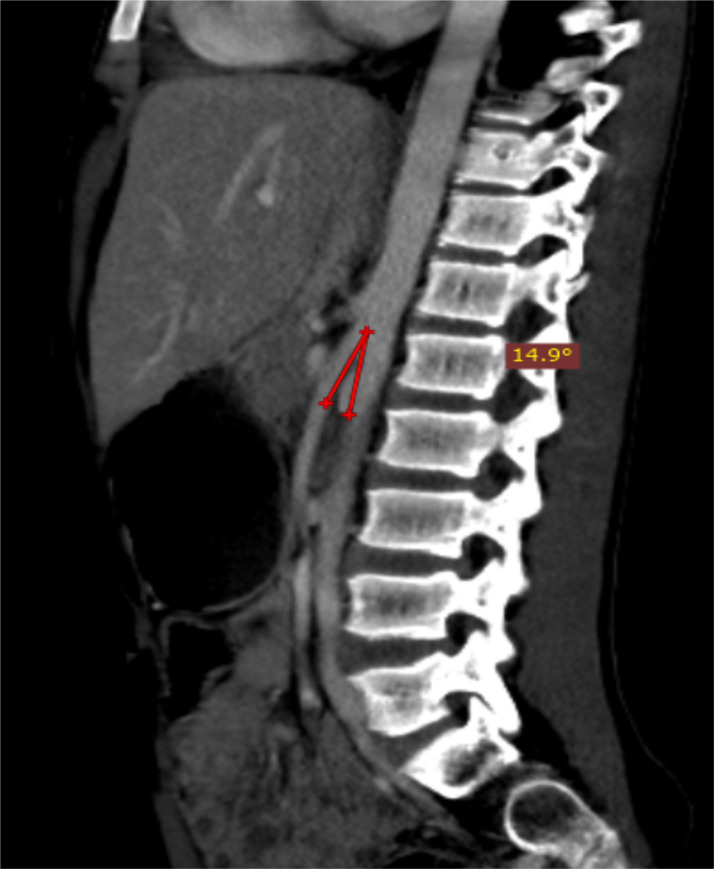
Abdominopelvic CT of a 12-year-old, known acute lymphocytic leukemia patient, on a post contrast mid sagittal image aortomesenteric angle (AMA) is 14.9^0^
